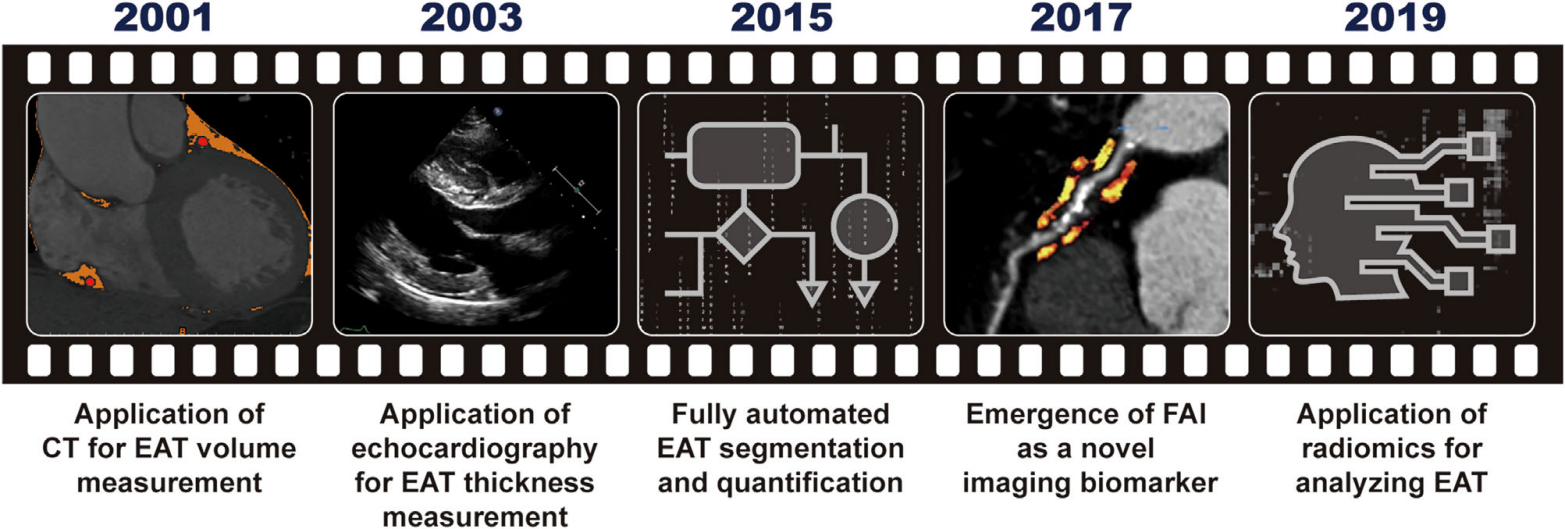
Milestones in the development of imaging techniques used in EAT‐related research. In 2001, Taguchi et al. first proposed a CT‐based measurement of EAT volume. In 2003, Iacobellis et al. first proposed using echocardiography to measure EAT thickness. Around 2015, algorithms for fully automated EAT segmentation and quantification emerged. In 2017, Antonopoulos et al. first proposed fat attenuation index of EAT as a novel imaging biomarker. In 2019, Oikonomou et al. first applied radiomics for analyzing EAT.
